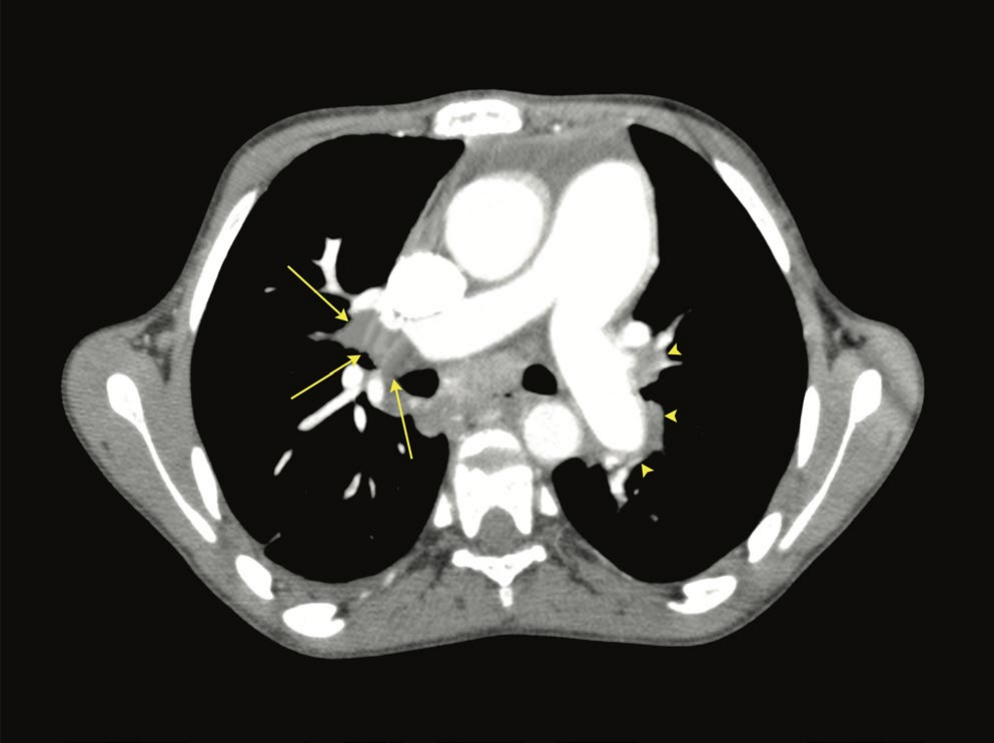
Chest CT angiography showing defects in the right (long arrows) and left (arrow heads) pulmonary arteries in keeping with bilateral chronic pulmonary thromboemboli
